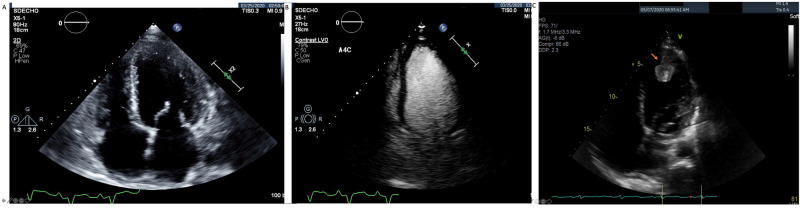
Left Ventricular Thrombus Formation in a Patient with Asymptomatic COVID-19 Initial transthoracic echocardiogram showed severely reduced left ventricular ejection fraction of 15-20% and global hypokinesis (A). There was no evidence of left ventricular thrombus in the contrast enhanced image (B). Subsequently, a large thrombus of 4.0 x 2.2 cm attached to the left ventricular apex is seen at the admission due to COVID-19 (C).
